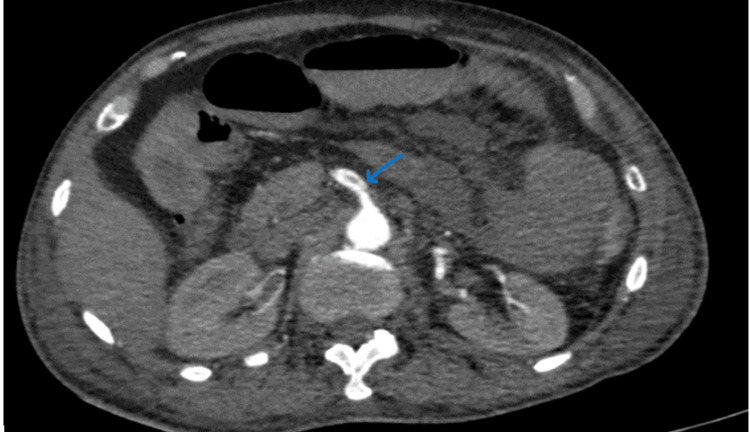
CECT of the abdomen and pelvis of the patient. CECT, contrast-enhanced computed tomography
